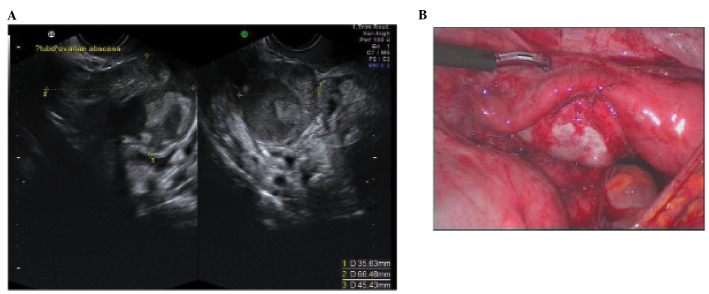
A tubo-ovarian complex. (A) Ultrasound appearances. (B) The same case at laparoscopy.
